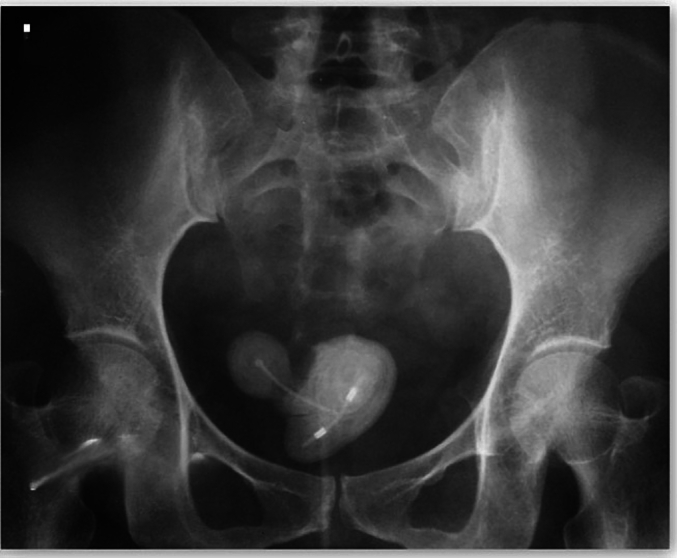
plain X-ray of the pelvis; a calcified bladder mass with the embedded intrauterine contraceptive device is observed.
